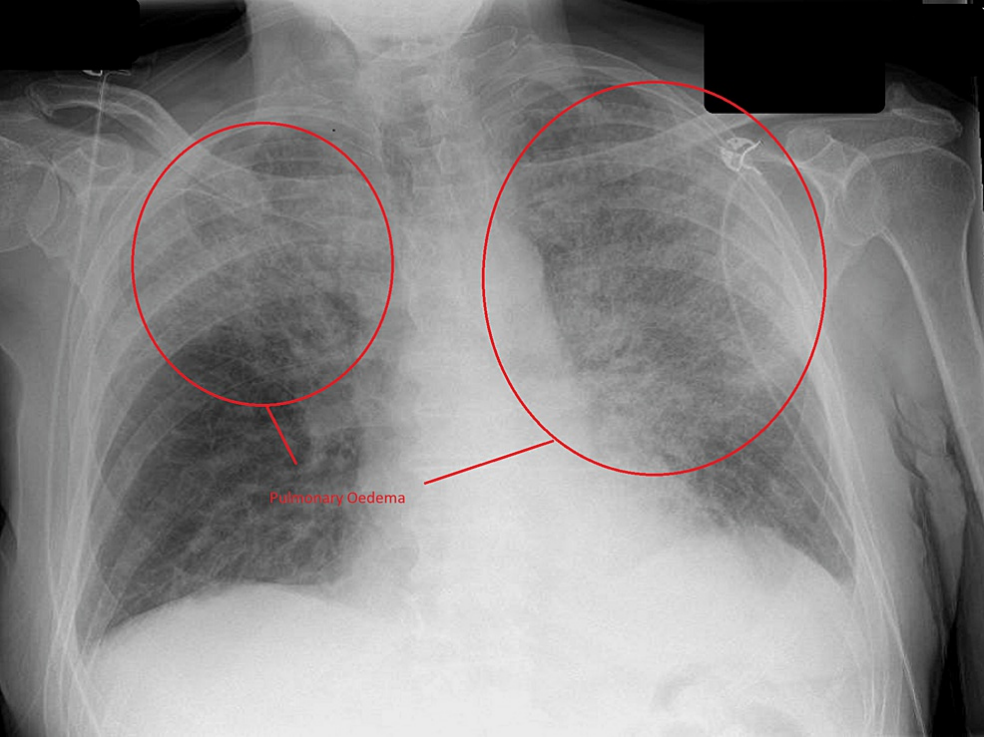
Chest X-ray. Chest X-ray with pulmonary oedema at the right upper, left upper, and middle zones.
